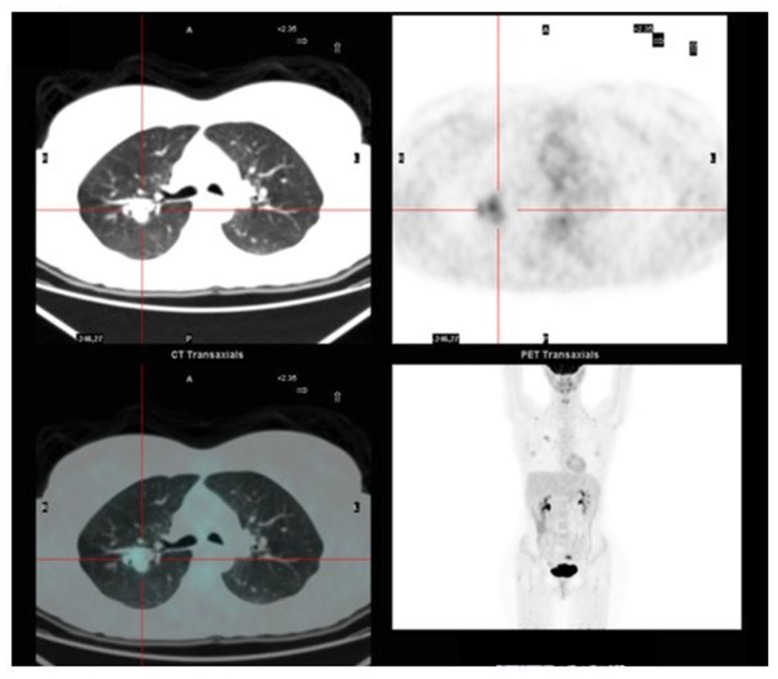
^18^F-FDG-PET of the pulmonary lesion.
